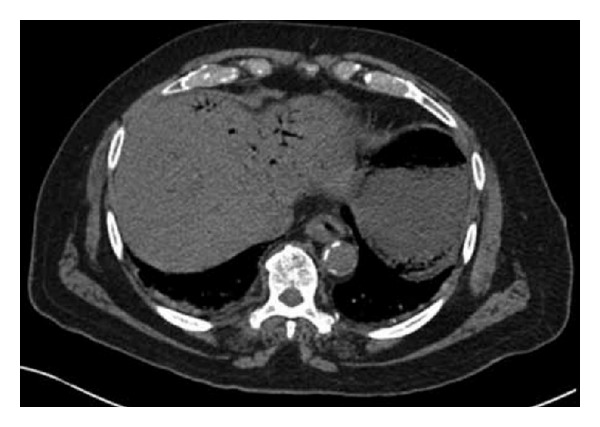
CT showing hepatic portal venous gas (Case 1).
